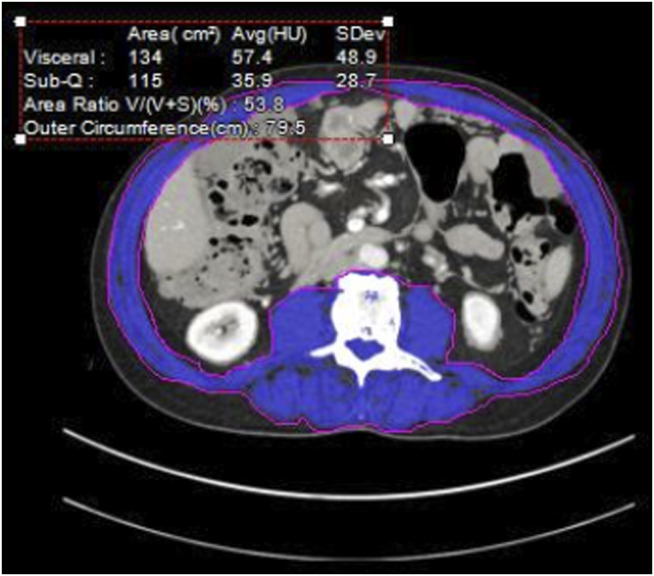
Cross sectional Abdominal CT at L3. Blue area—quantified muscle [3].
